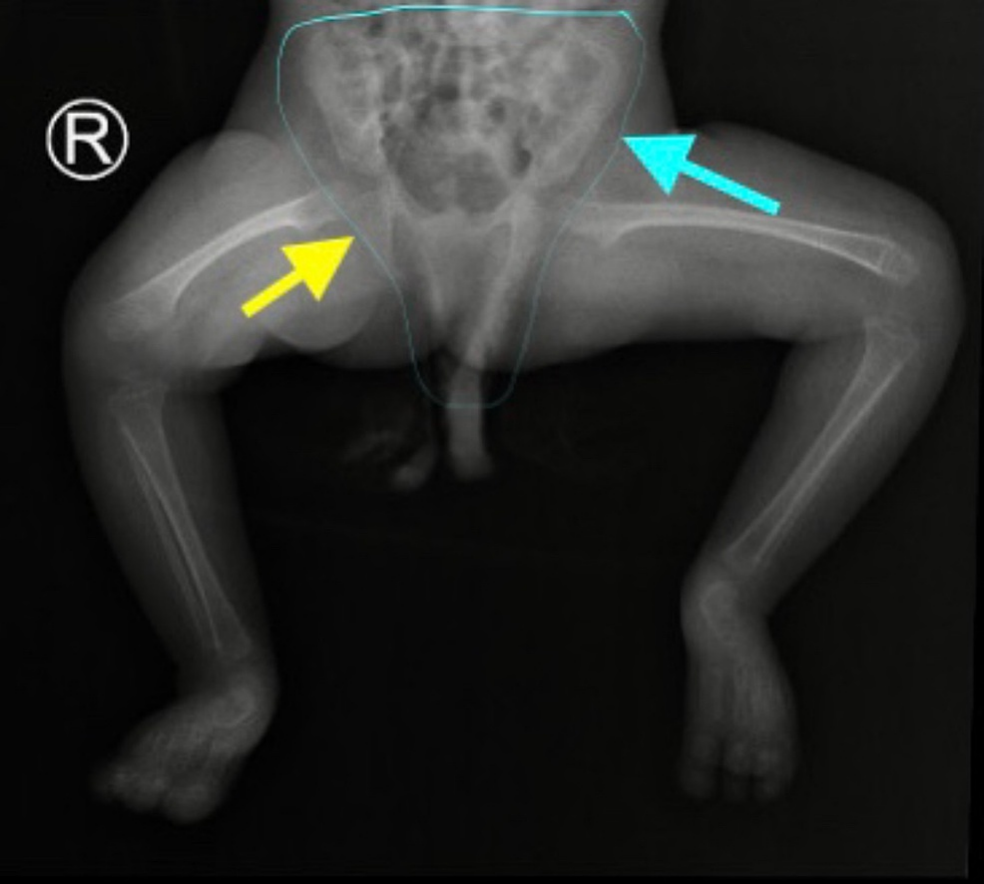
X-ray of the lower limbs (part of the skeletal survey): anteroposterior view showing a high and narrow hip (blue arrow and line) and a flat acetabulum (yellow arrow).
